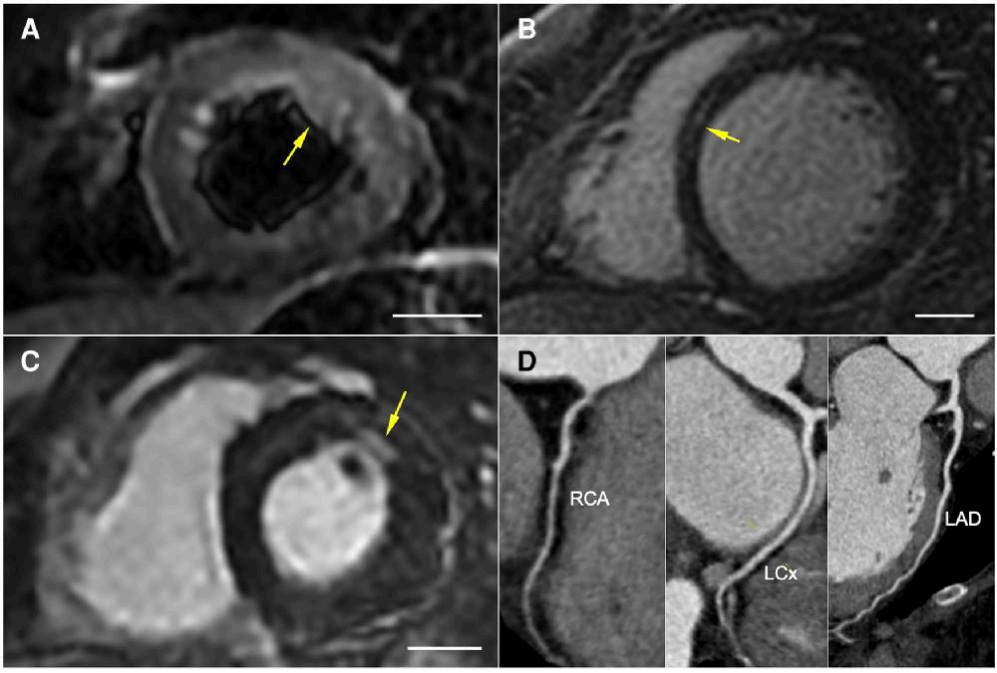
Cardiac MRI abnormalities in patients after COVID-19. Representative images showing cardiac MRI abnormalities (arrows) detected in patients after COVID-19 infection: (*A*) increased T2-weighted oedema signal in the mid-anterolateral segment; (*B*) linear mid-wall LGE in the basal septum; and a (*C*) small focus of basal anterior sub-endocardial LGE in a patient with (*D*) no epicardial coronary artery disease on CTA. Scale bar = 20 mm.
